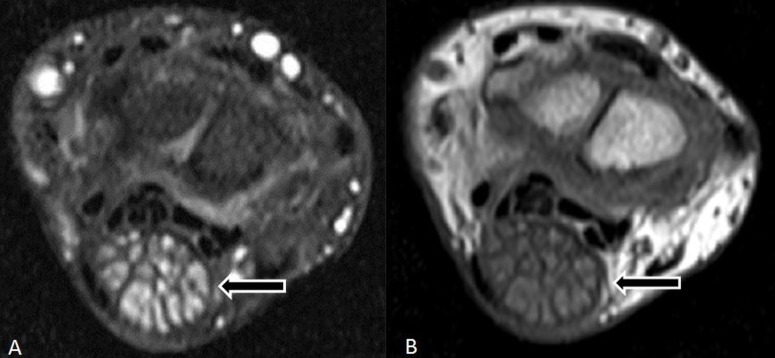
A) Axial T1-weighted FS + C image and B) Axial T2-weighted image of wrist shows significantly enlarged median nerve with hypertrophied nerve fascicles (arrow) stretching the flexor retinaculum, causing the carpal tunnel syndrome.
